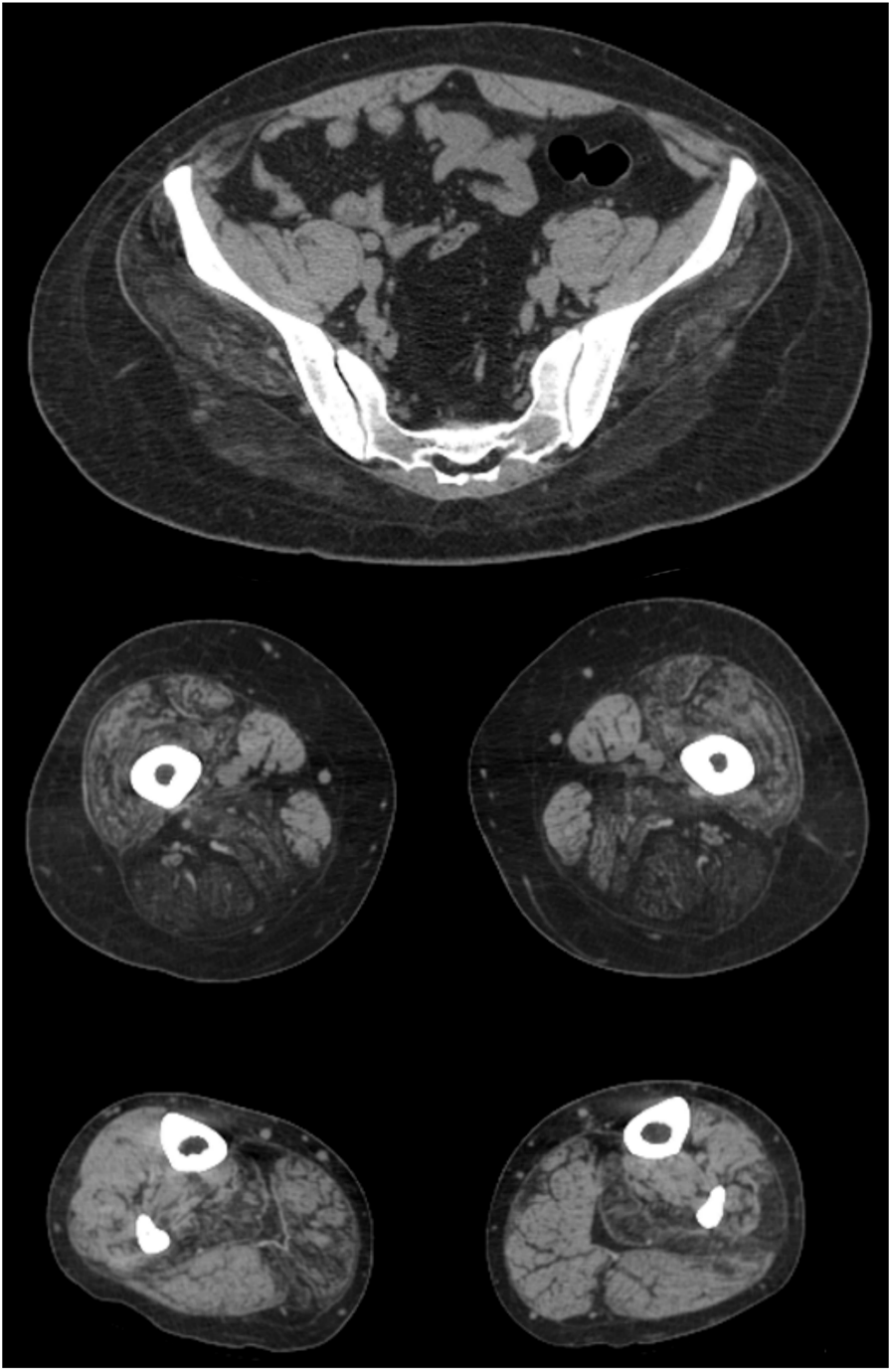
Computed tomography of the pelvis, thighs and legs. At the pelvis there is advanced gluteus maximus, gluteus medius, and gluteus minimus involvement. At the thigh, there is advanced biceps femoris, semitendinosus, semimembranosus involvement; severe vastus lateralis, vastus medialis, vastus intermedius, and adductor magnus involvement, with relative preservation and hypertrophy of sartorius and gracilis. At the leg, there is asymmetric muscle involvement with advanced right gastrocnemius medialis, left peroneus, bilateral soleus involvement, compared to tibialis anterior muscle sparing.
